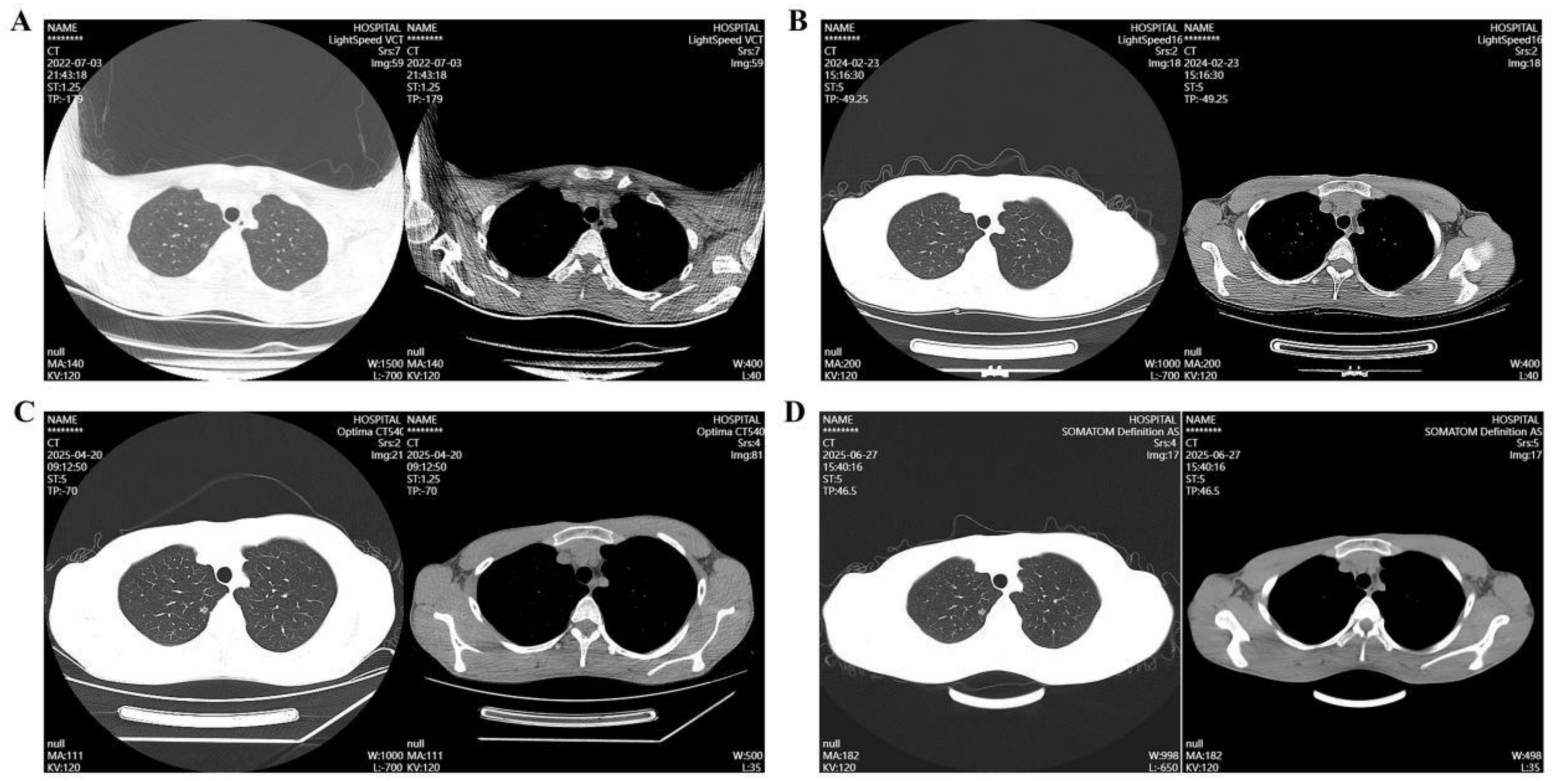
Chest CT scan showing a nodule in the right upper lung of the patient during follow-up
